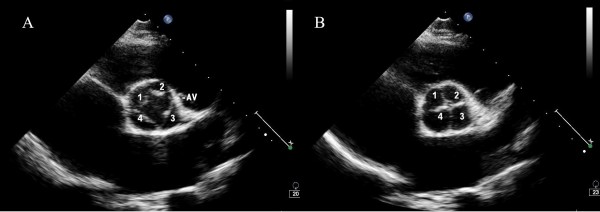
Echocardiography of a 20-year-old asymptomatic boy with quadricuspid aortic valve (QAV). Short axis view during systole (A) and diastole (B) revealed four equal cusps of aortic valve.
